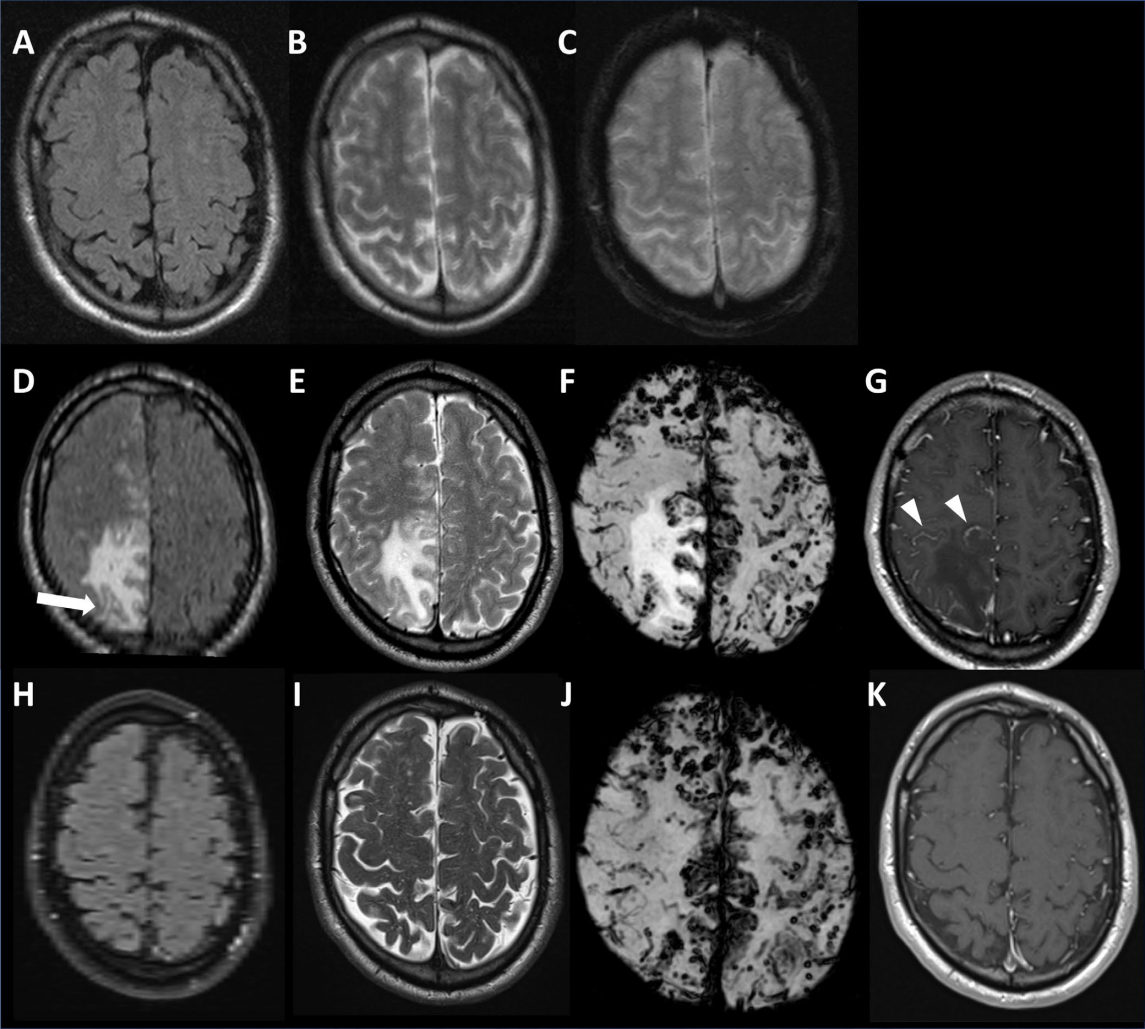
Evolution of the imaging features 7 years before (A–C), during (D–G) and after (H–K) the inflammatory episode. During the episode, the right parietal lobe demonstrates focal swelling, vasogenic edema on FLAIR (D) and T2w (E), adjacent sulcal hyperintensity (arrowhead) on FLAIR (D) and leptomeningeal enhancement (arrowhead) on contrast enhanced T1w (G). Note complete resolution (H–K) after 6 months follow‐up. T2* (C) and SWI (F, J) depicting the hemorrhagic markers on corresponding slices.
